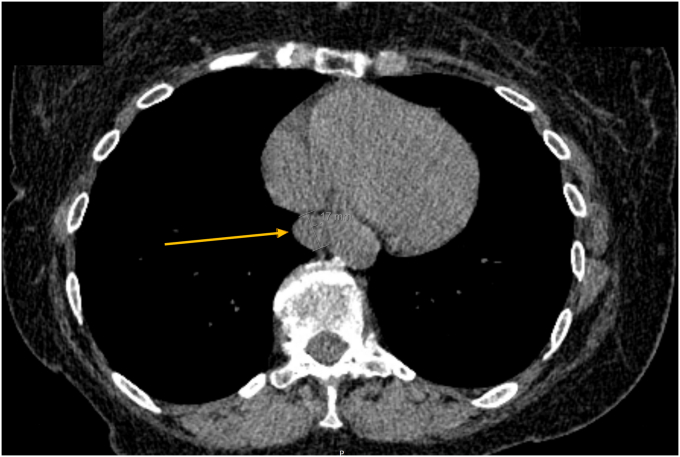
Noncontrast computed tomography of the chest showing a round soft-tissue lesion along the distal esophagus with a mean diameter of 1.7 cm (*yellow arrow*).
